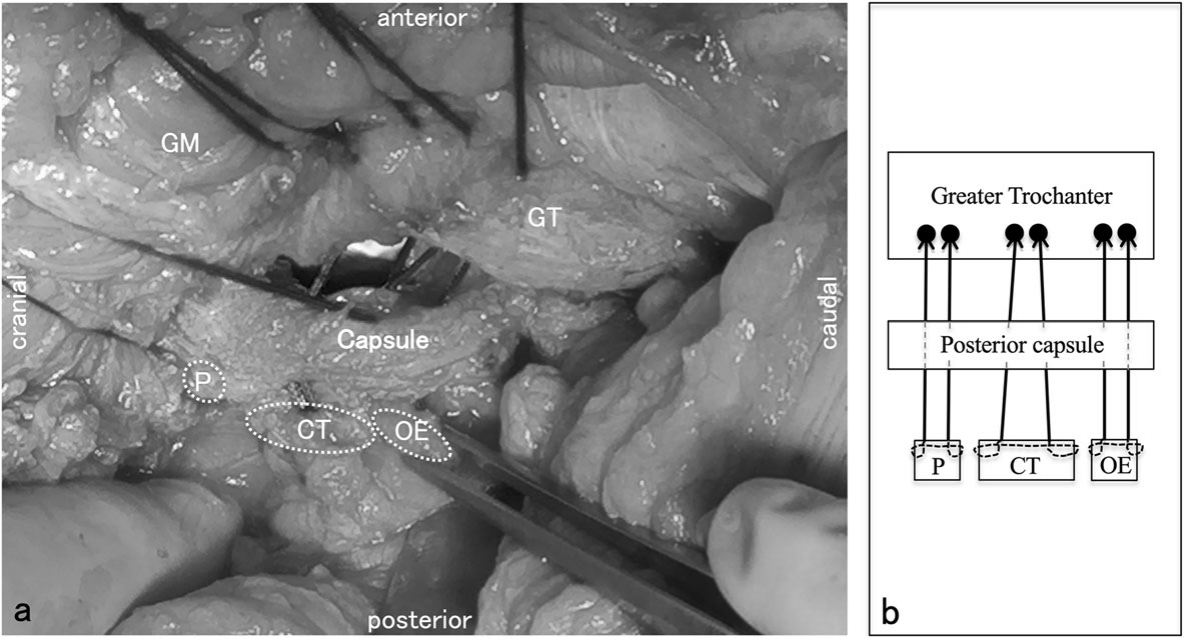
Intraoperatively, the hip joint is placed in the neutral position. GM, gluteus medius; GT, greater trochanter; P, piriformis; CT, conjoined tendon; OE, obturator externus. a Showing obturator externus and other posterior soft tissues. b The short external rotators and joint capsule were sutured to the posterior insertion of the greater trochanter using three non-absorbable sutures
